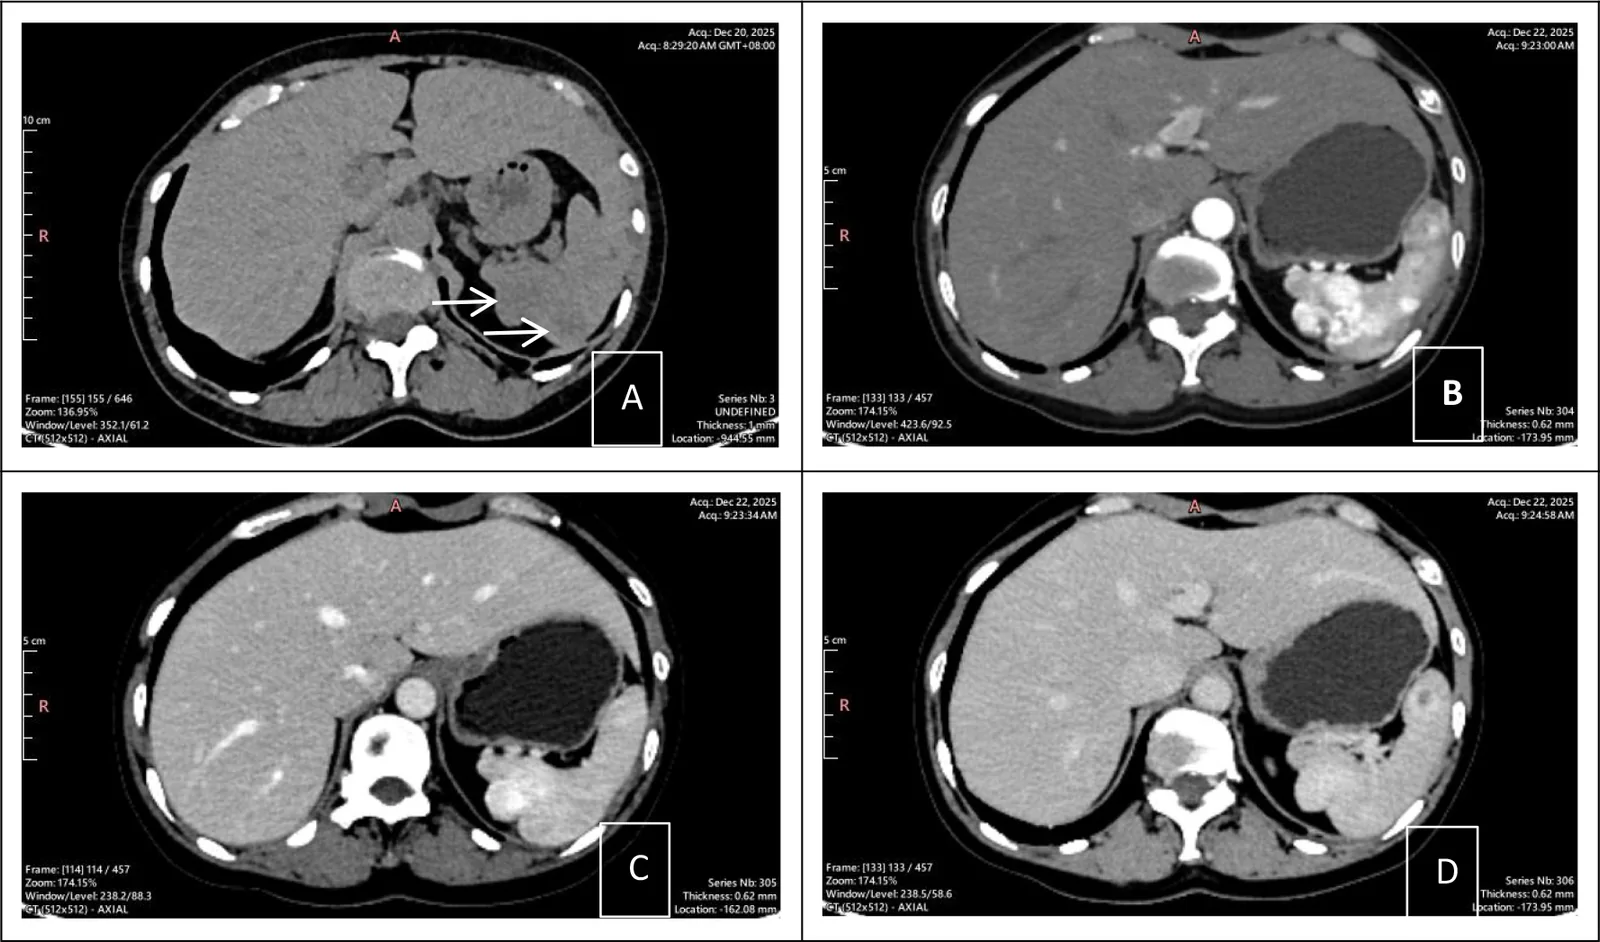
Abdominal CT imaging findings. (A) Non-contrast CT of the abdomen demonstrates multiple slightly low-density nodules in the spleen (arrows), some of which protrude beyond the splenic contour. (B–D) Contrast-enhanced abdominal CT [arterial phase (B), portal venous phase (C), delayed phase (D)] shows progressive centripetal fill-in enhancement of the splenic lesions, which gradually become isodense with the surrounding splenic parenchyma.
